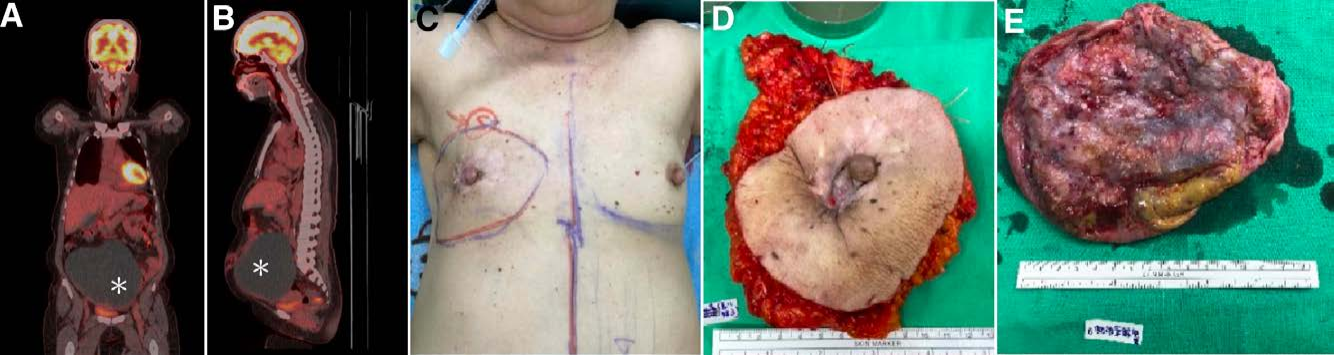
CT images and breast tumor presentation. (A) Coronal and (B) sagittal views of the abdominal mass. (C) Presurgical contouring of breast cancer. (D) Breast cancer lesion. (E) Ovarian tumor. *: right ovarian tumor, size: 17.2 cm × 14.3 cm × 0.3 cm.
